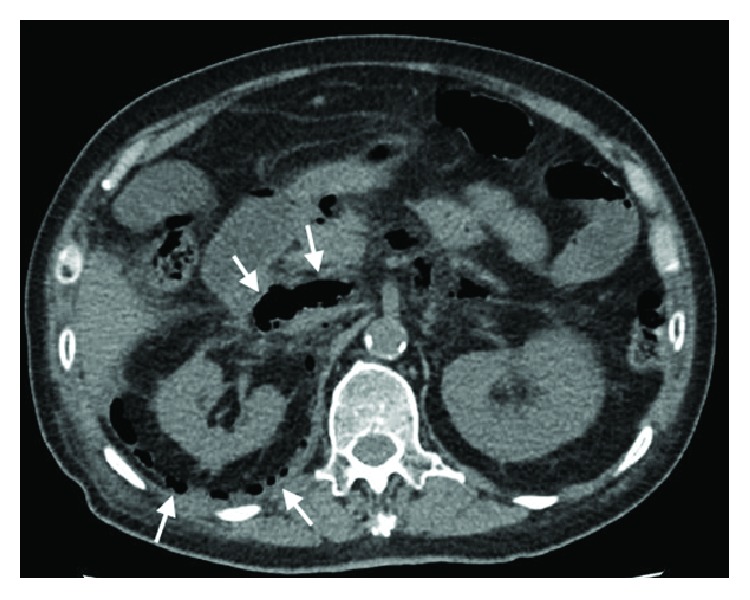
CT scan of abdomen showing pneumoperitoneum at region of 2nd and 3rd part of the duodenum with retroperitoneal air involving the right perinephric region.
